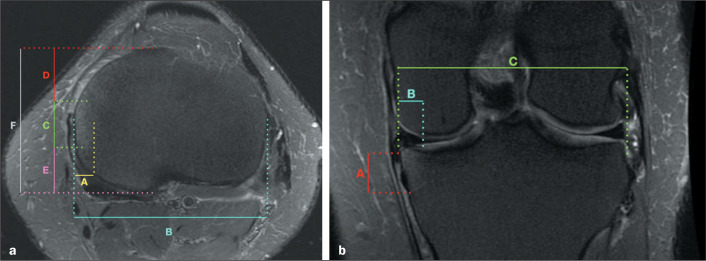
T2-weighted MRI with fat saturation, showing an example of bone edema
